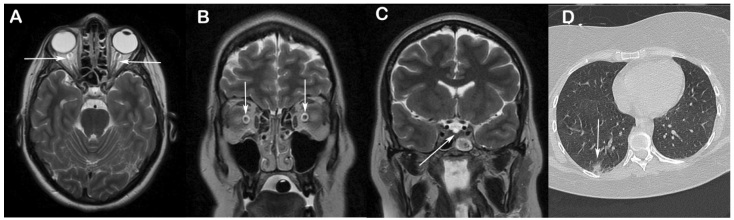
(A and B) T2 axial and coronal magnetic resonance imaging scan shows prominent subarachnoid space around the optic nerves and their vertical tortuosity (arrows); (C) Coronal T2 magnetic resonance imaging scan of the selar region demonstrates compression of the upper portion of the pituitary gland by the selar diaphragm (arrow). (D) Chest computed tomography shows round peripheral ground-glass opacities in the right lung (arrow).
